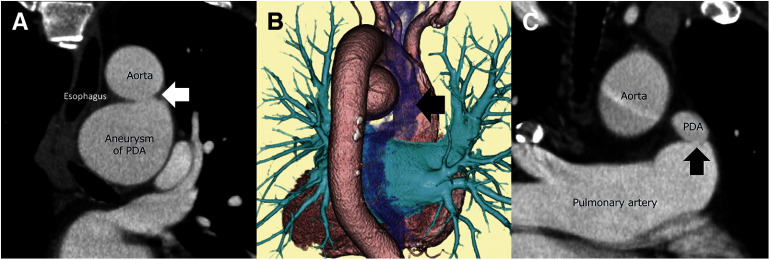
Fig. 1 Preoperative contrast-enhanced CT image. (A) PDA aneurysm compressing on the esophagus, and the aortic side orifice (white arrow) with a diameter of 14 mm. (B) The aneurysm located under the aortic arch and aneurysmal sac measured 57 × 50 mm. It exerted pressure on the esophagus (black arrow, blue colored: esophagus). (C) The pulmonary side orifice (black arrow) with a diameter of just 4 mm. CT: computed tomography; PDA: patent ductus arteriosus.
